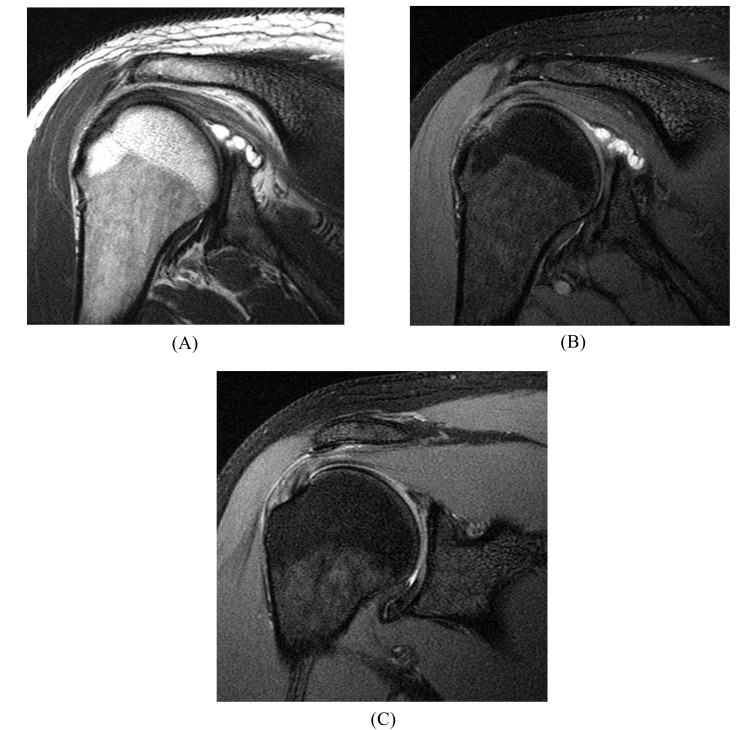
SLAP tear in a 39 year-old patient with a history of a negative c-spine MRI. Lobulated paralabral cyst at the posterosuperior glenoid rim due to a labral tear on (A) a coronal intermediate-weighted image and (B) a coronal fat-suppressed T2-weighted image. (C) At the adjacent superior labrum near the biceps anchor, there is subtle, laterally curved, linear signal in the labrum indicative of a SLAP tear (without arthrography).
